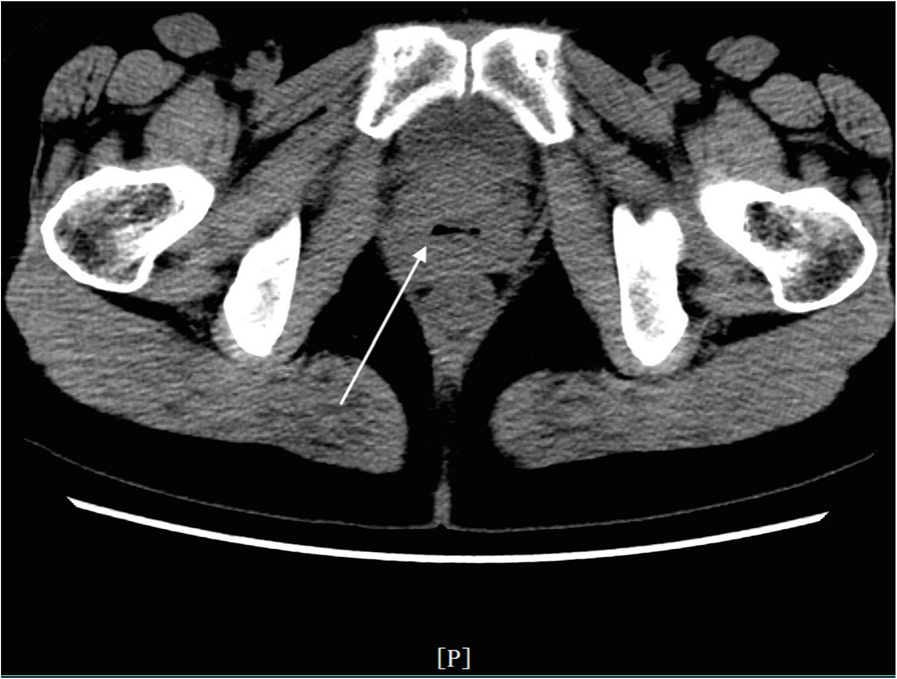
CT findings of ROCLC. Patient presented with persistent abdominal pain for a period of 2 hours which started after intercourse; HCG test was negative and there were no signs of pelvic hemorrhage. The single arrow indicates the air shadow in the cervix
